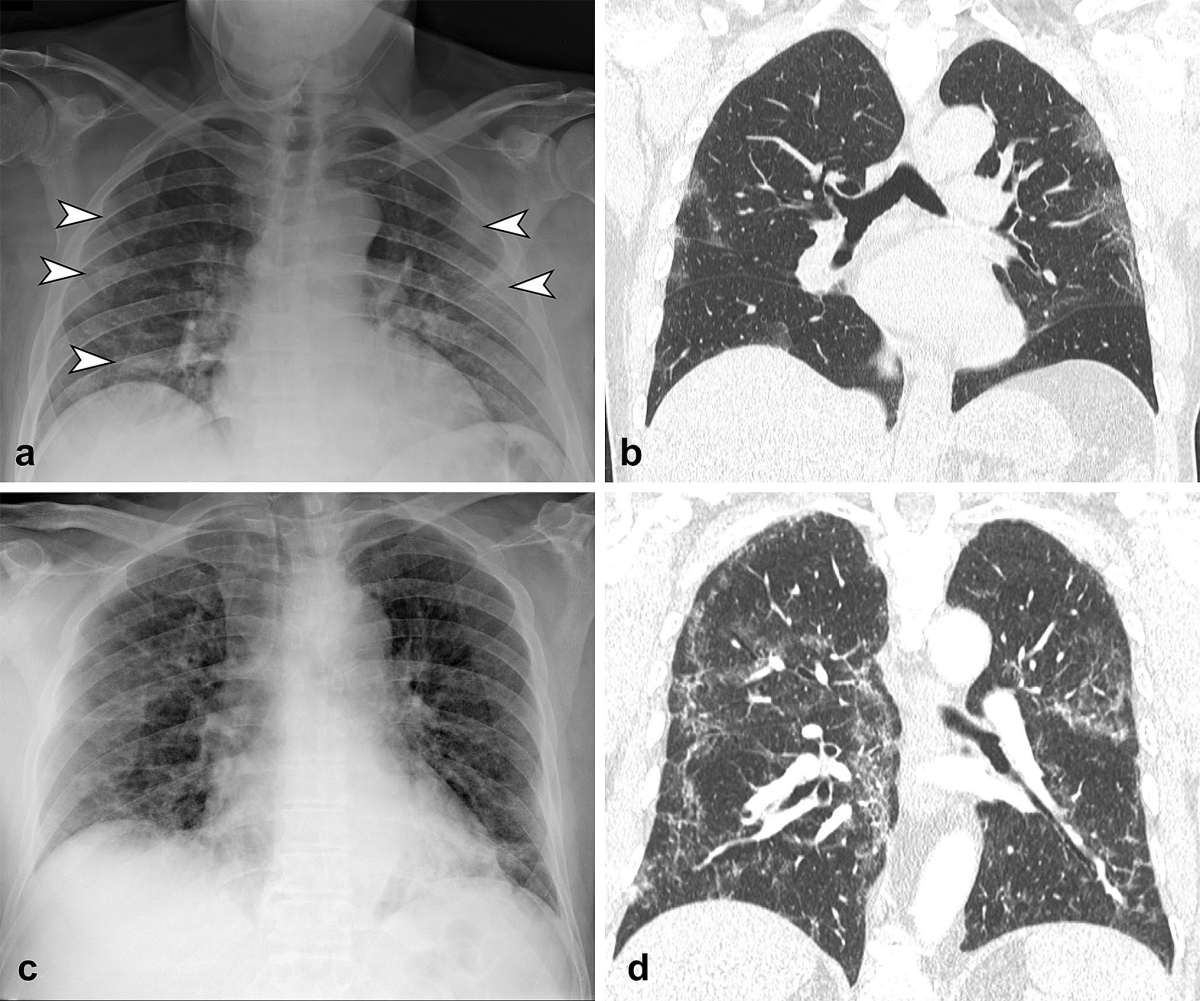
a CXR in antero-posterior view shows bilateral ground glass opacities (arrowheads) with peripheral distribution, involving middle-lower zone of the lungs. b Coronal reformatted CT image confirmed the CXR findings. c CXR in antero-posterior view shows bilateral reticular pattern with diffuse distribution on both axial and longitudinal plane. d Coronal reformatted CT image confirms the presence of diffuse interstitial involvement with reticular pattern
